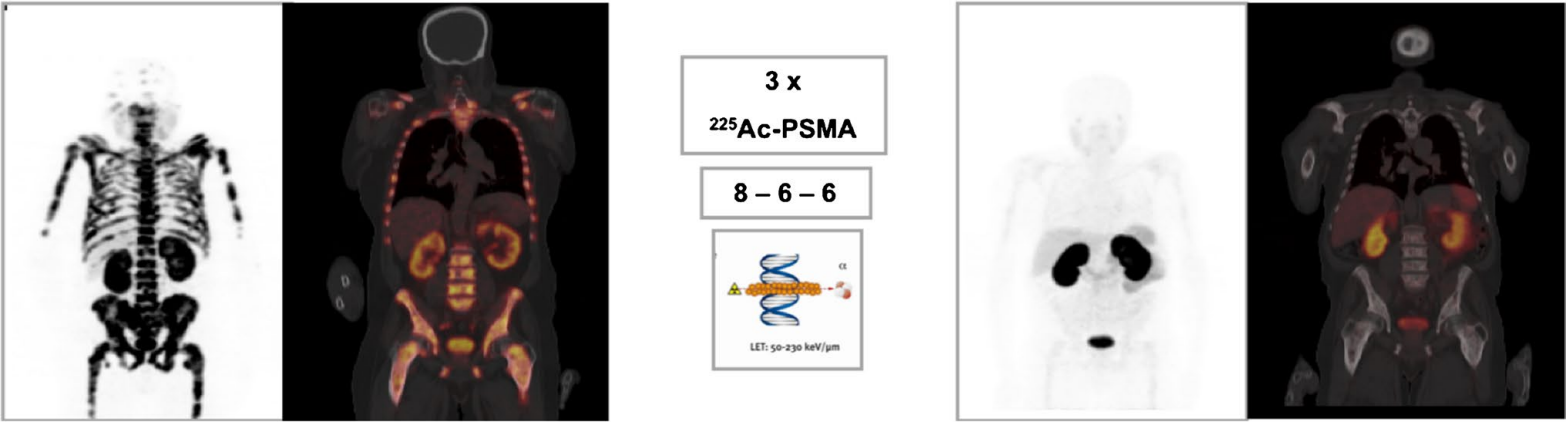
A 67-year-old-male with extensive skeletal metastases, Gleason score = 4 + 5. He was treated with 225Ac-PSMA-617 following disease progression on conventional therapy. The bone marrow remained stable after treatment
